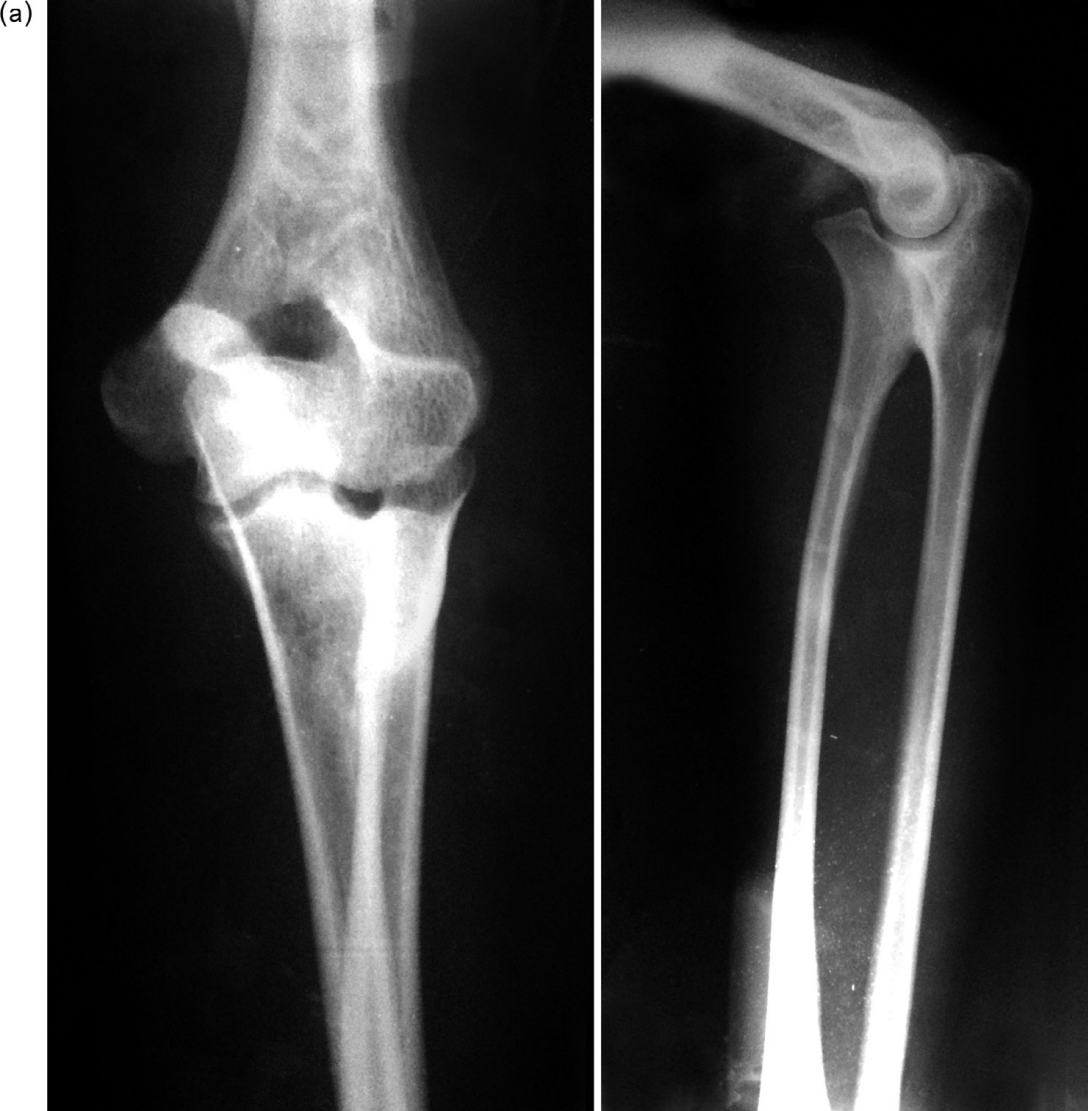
(a) and (b) Bilateral preoperative anteroposterior and lateral radiographs of elbows (Case no. 1), showing proximal radioulnar synostosis with radial head displaced anteriorly consistent with a type-IV deformity [1].
